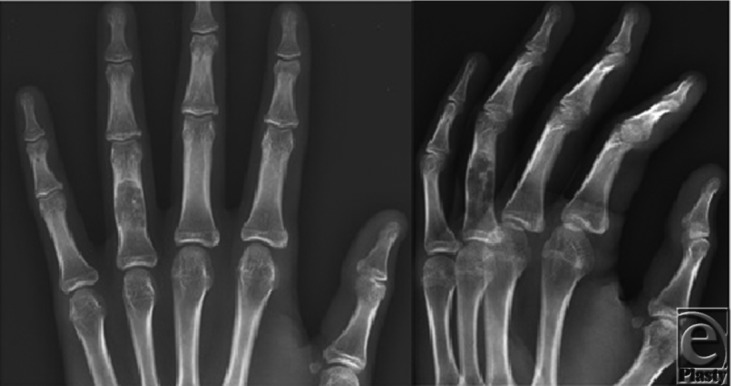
Radiographs of the left hand with an expansile lytic lesion within the midshaft of the ring finger proximal phalanx with endosteal scalloping and thinning of the surrounding cortex.
